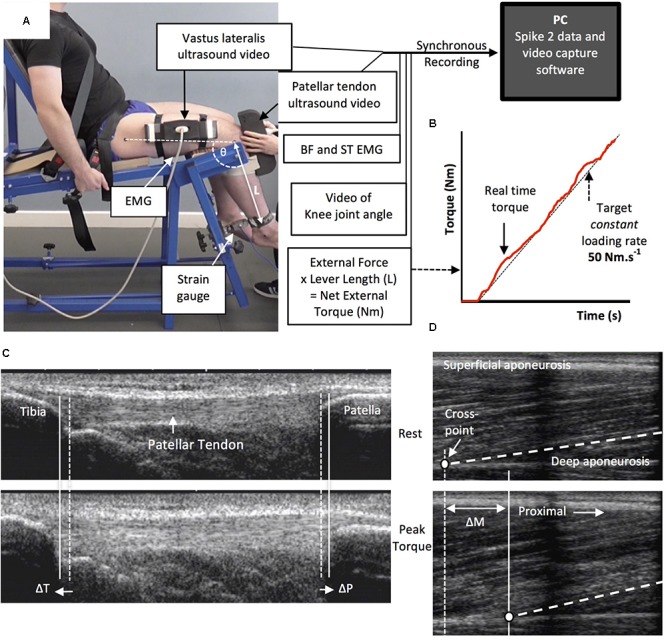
The experimental set-up and ultrasound images during the ramp contractions. Participants were tightly fastened to a rigid isometric strength-testing chair with resting knee and hip angles of 115° and 126°, respectively (A). Unilateral knee extension torque, video of the knee joint angle, antagonist muscle [biceps femoris (BF), semitendinosus (ST)] surface electromyography (EMG) and ultrasound video images were synchronously recorded during constant-loading rate isometric ramp knee extensor contractions (example in B). Ultrasound images are of the patellar tendon (C) and vastus lateralis muscle (D) at rest (top) and at peak ramp torque (bottom) and indicate the measurement of patellar tendon (tibia-patella apex displacement, ΔT + ΔP) and tendon–aponeurosis complex (vastus lateralis muscle fascicle-deep aponeurosis cross point proximal displacement, ΔM) elongation.
